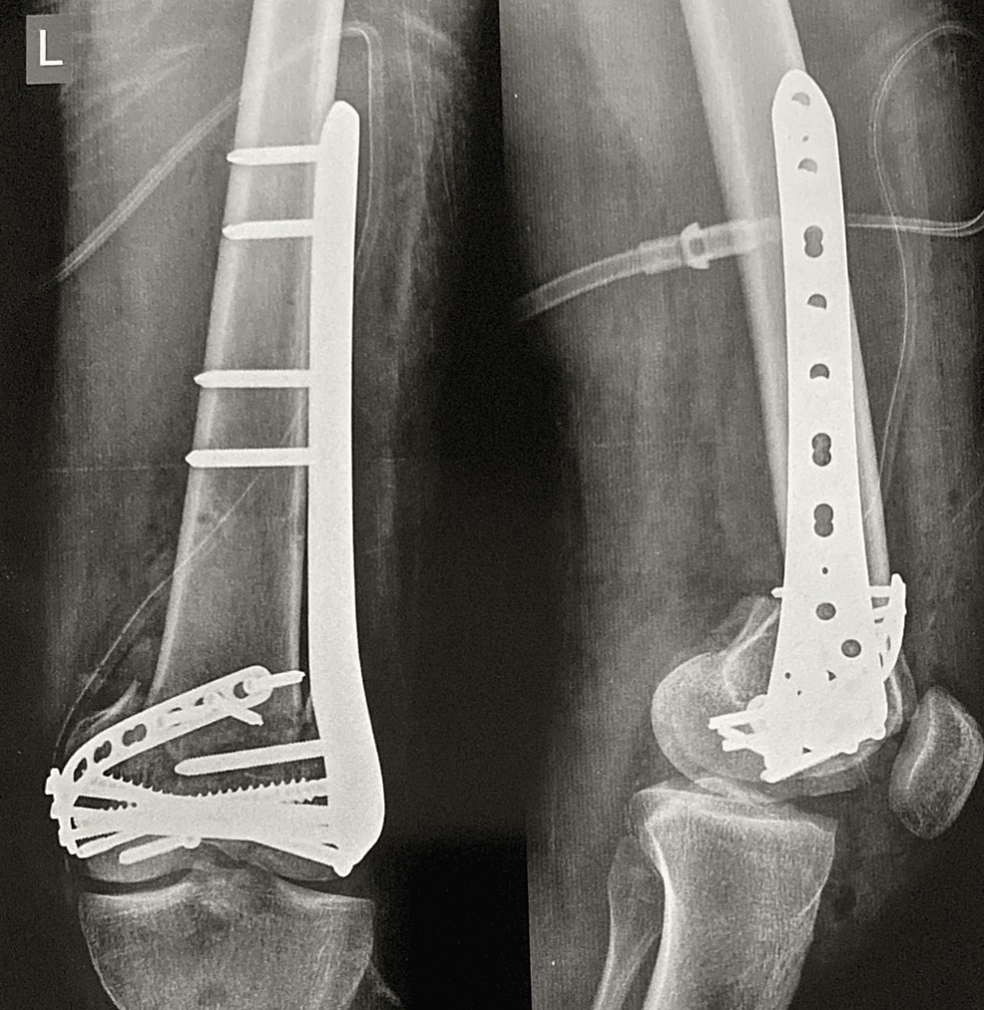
Postoperative radiograph after fixation of the fracture with distal femur locking plate over the lateral aspect and T-buttress plate over the medial aspect
